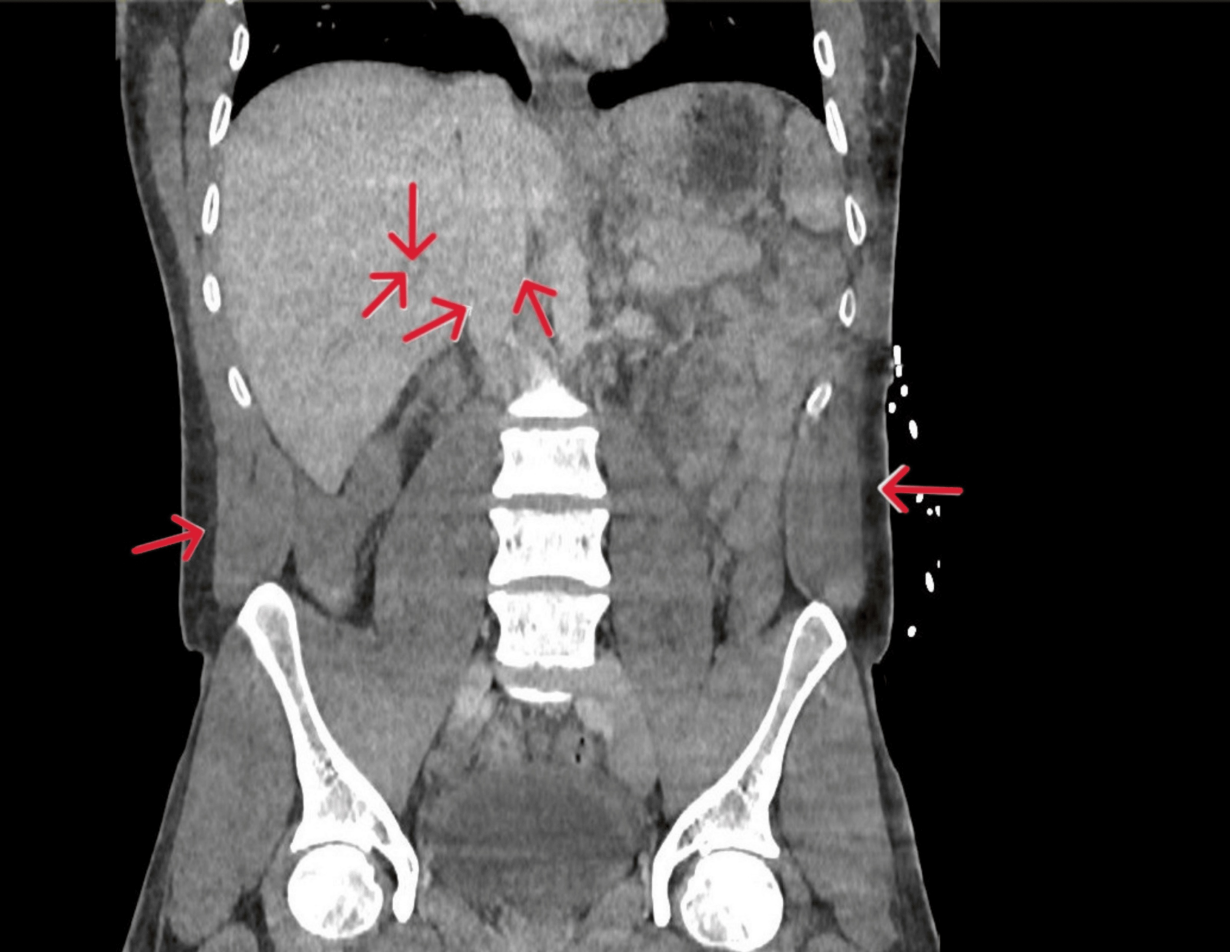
CT abdomen Day 0 coronal section showing prominent hepatic veins and inferior vena cava, suggesting hepatic venous congestion. Generalised oedema of the intra-abdominal and subcutaneous fat. Red arrows indicate prominent hepatic veins, the inferior vena cava, and generalised oedema of the subcutaneous fat.
